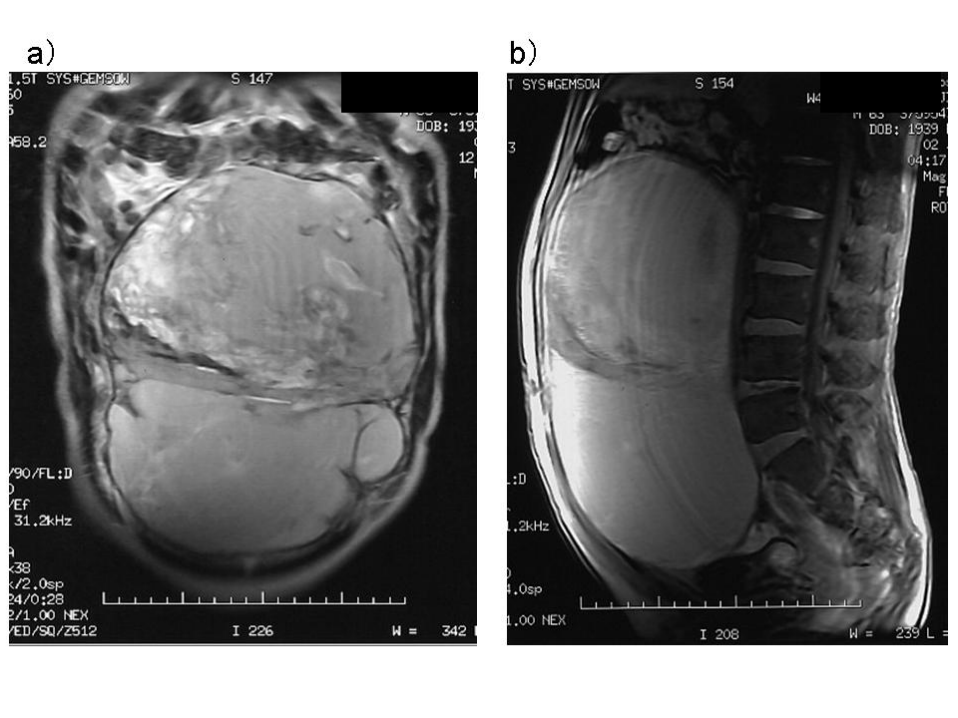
MRI detected a thick septum in the tumor center, which divided the tumor into the upper and lower regions. T2-weighted imaging showed coexistence of areas with high and low signal intensity. (a) coronal T2-weight imaging (b) sagittal T1-weight imaging.
